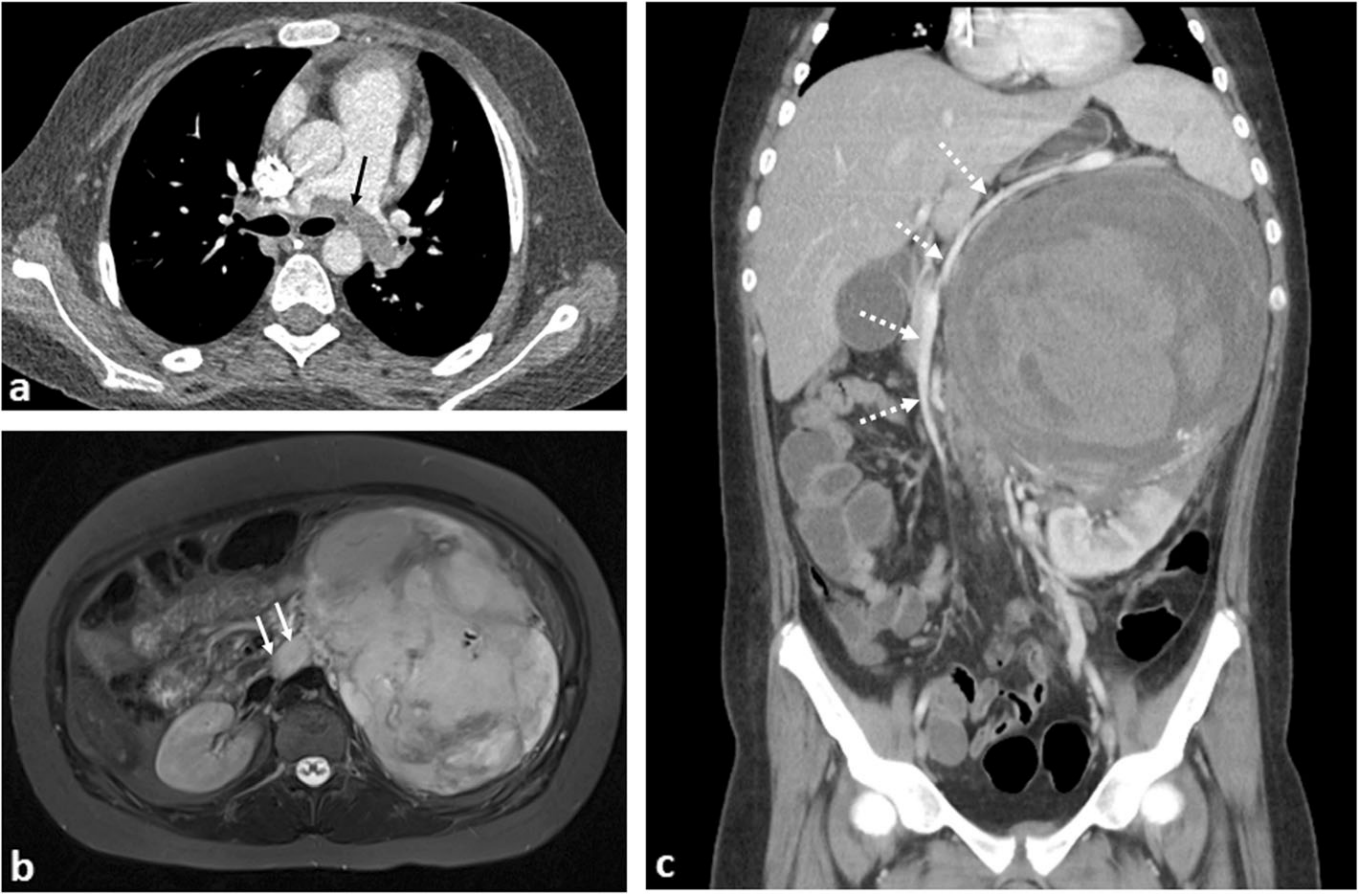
A 13-year-old girl with left-sided Wilms’ tumour and saddle pulmonary embolus. a Axial computed tomographic pulmonary angiogram (CTPA) demonstrates a saddle pulmonary embolus occupying the right and left pulmonary arteries (black arrow). b Axial T2-weighted fat-saturated MR image reveals a left renal mass with tumour thrombus occupying the entire left renal vein (white arrow). c Coronal contrast-enhanced CT image of the abdomen shows the heterogeneously enhancing left renal mass, with internal haemorrhage. There is right-sided displacement of the IVC with the splenic vein (dashed arrows) stretched and draped over the mass
